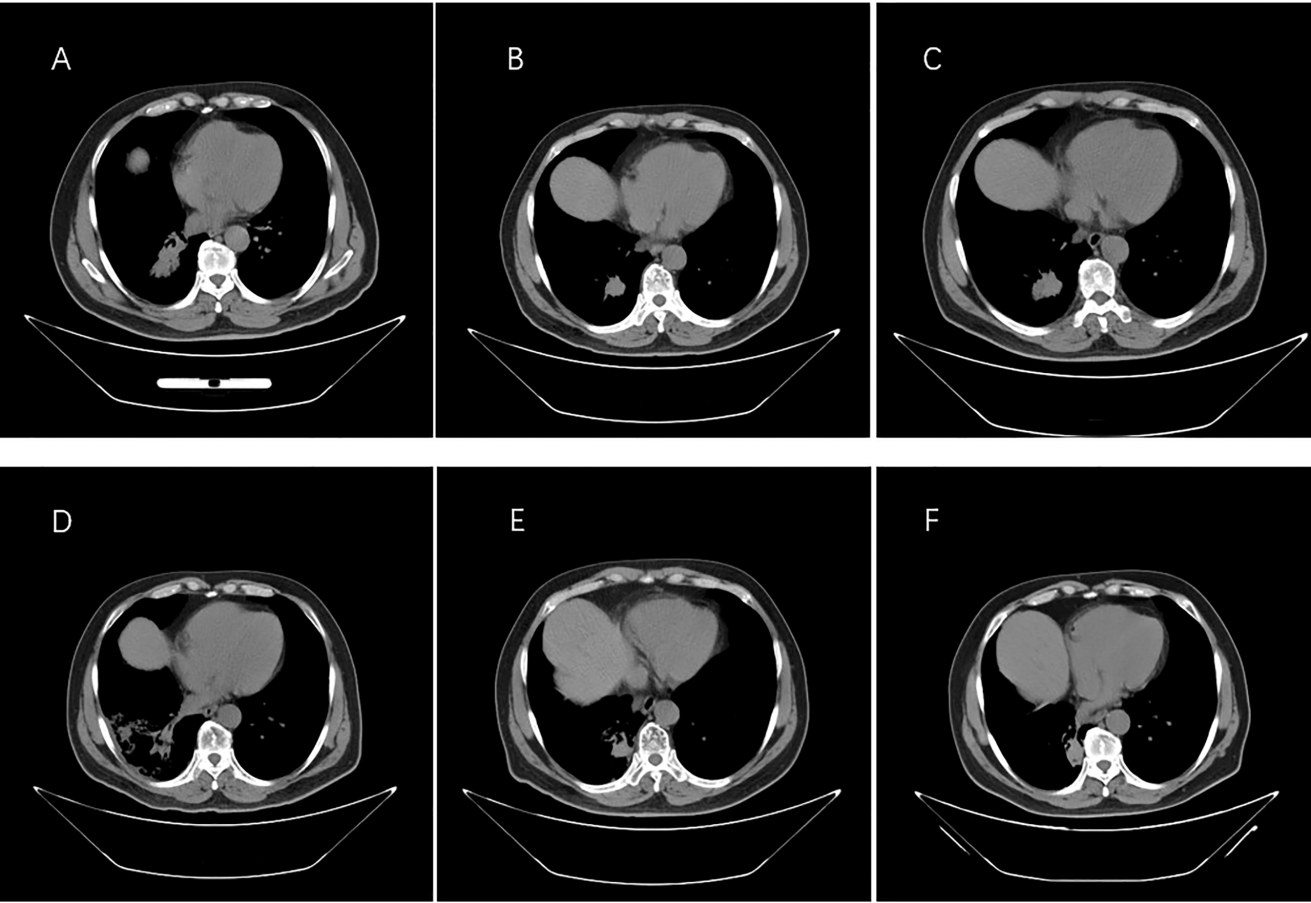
Images from computed tomography showing the tumor in the right lung in response to therapy. (A) Before therapy, imaging was performed on 30 December 2016. (B) Six months after taking gefitinib on 18 August 2017. (C) Resistance progresses on 20 January 2018. (D) After the radiotherapy on 5 June 2018. (E) One year after taking Osimertinib on 18 February 2019. (F) Last check on 20 May 2022.
